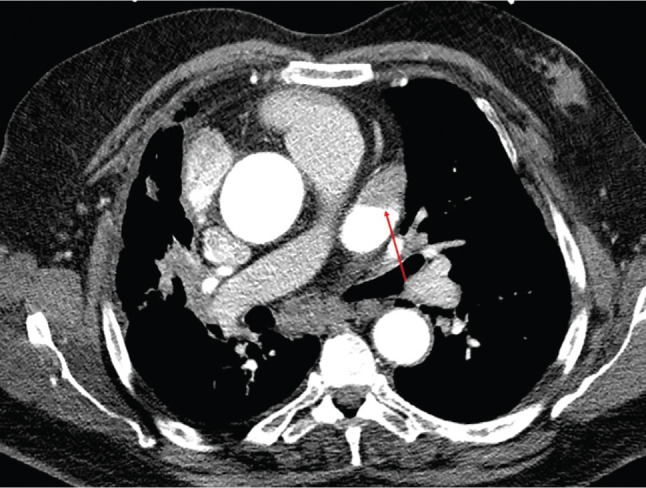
A patient with persistent AF and a CHA_2_DS_2_-VASc score = 4 found to have a filling defect on cardiac CT suggestive of LAA thrombus. The red arrow points out the LAA thrombus.
